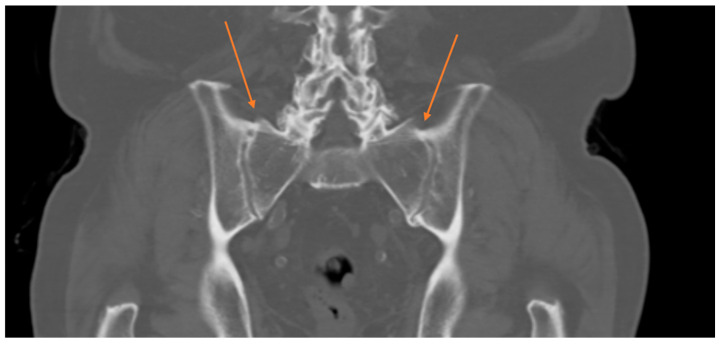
Coronal CT of the pelvis showing signs of spontaneous sacroiliac-joint fusion cranial and anterior of the SI joint (arrows) making posterior stabilization of the pelvis unnecessary despite wide resection of the symphyseal region.
